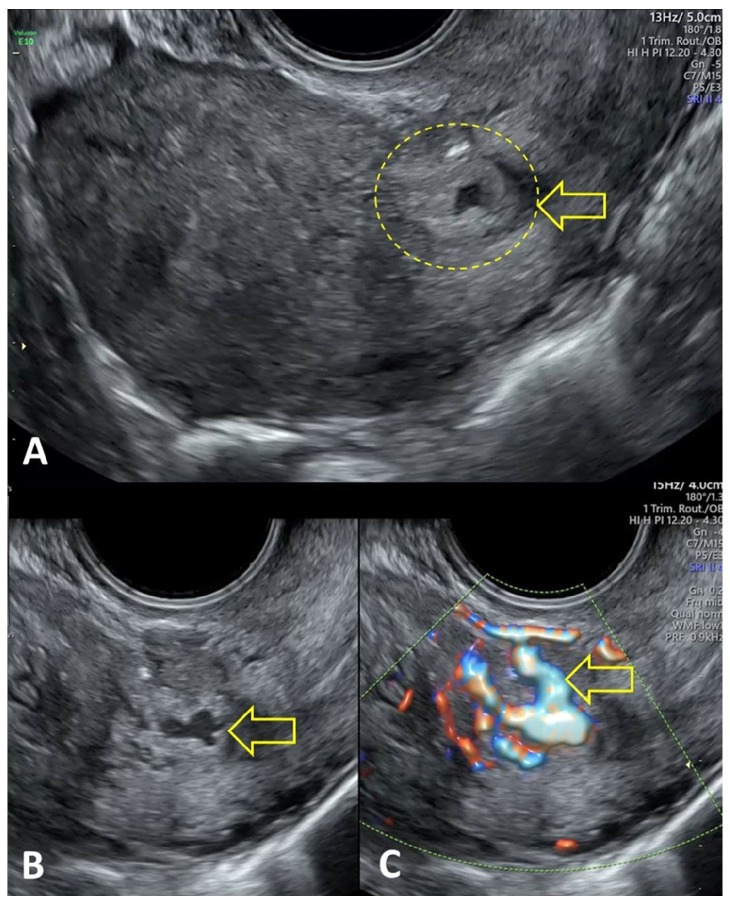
Transvaginal ultrasound and color Doppler findings characteristic of acquired uterine arteriovenous fistula/malformation (AVF/AVM). (A,B) Gray-scale transvaginal ultrasound showing a heterogeneous myometrium with clusters of serpiginous anechoic channels adjacent to a previous cesarean-section scar (indicated by a yellow arrow). (C) Color Doppler demonstrating intense aliasing and a mosaic pattern of turbulent, high-velocity, low-resistance flow consistent with arteriovenous shunting (indicated by a yellow arrow). AVF, arteriovenous fistula; AVM, arteriovenous malformation.
